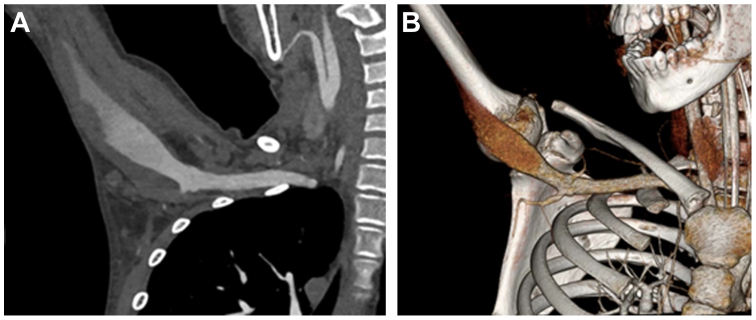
(A) Preoperative computed tomography angiography. (B) Preoperative reconstruction.
